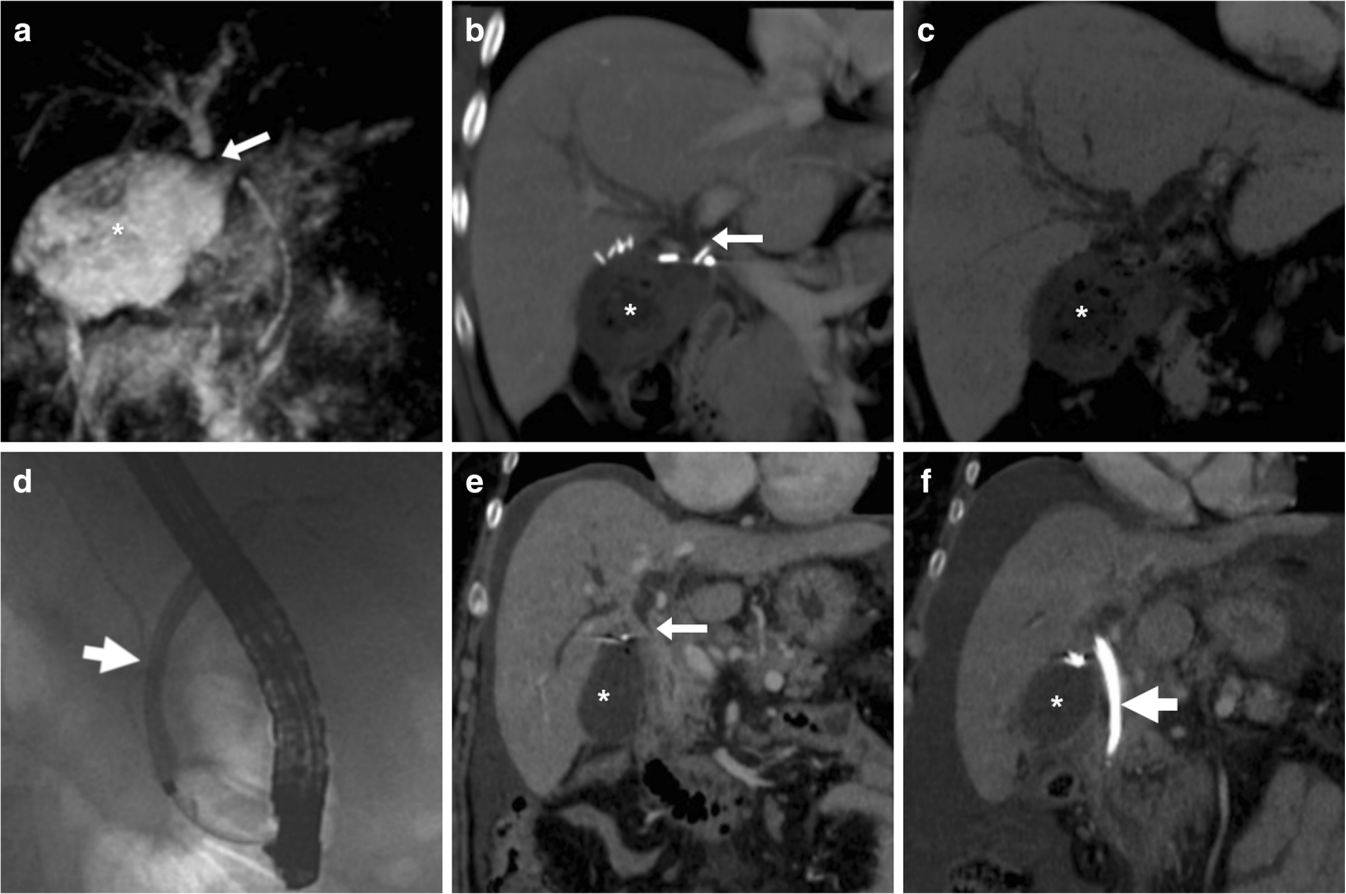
Two patients with biliary obstruction from iatrogenic early post-laparoscopic cholecystectomy biliary injuries. a–d On the 5th postoperative day, MRCP (a) showed usual postoperative collection at gallbladder fossa (*), dilated intrahepatic ducts and proximal CBD with abrupt termination (*arrow*) and normal choledochus distally to the discontinuity. Additional focused contrast-enhanced CT image (b) better showed the metallic clips and the MIP image (c) depicted the dilated intrahepatic bile ducts. The dilated common hepatic duct measured 14 mm in length, consistent with a Bismuth type II injury. After ERCP (d) confirmation of impassable obstruction, reoperation was required to remove the misplaced clips. (Adapted with permission from ref. [44].) e, f On the 12th postoperative day, CT (e) showed ascites, fluid collection in the surgical bed (*) and a CBD stricture (*arrow*) attributed to probable thermal injury, which was treated endoscopically with the positioning of a stent (*thick arrow* in post-procedural CT, f)
